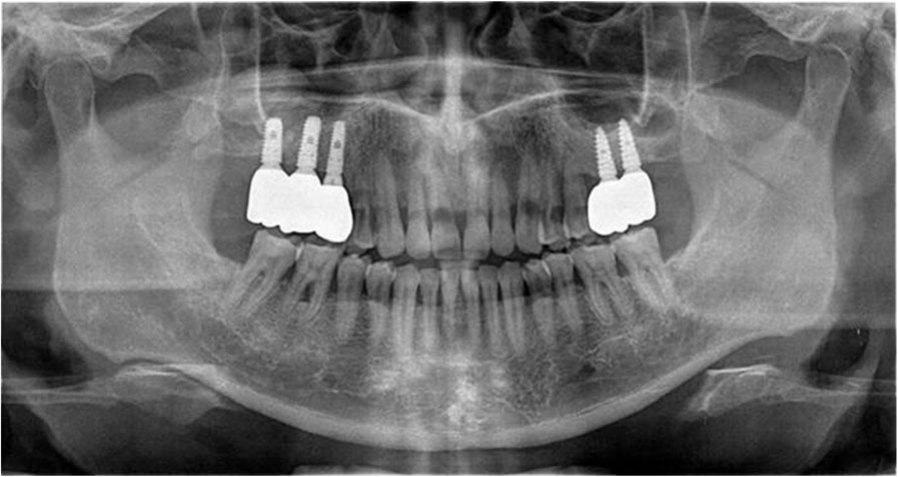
Panoramic radiograph 3 years and 9 months after sinus bone graft. There were no postoperative complications
